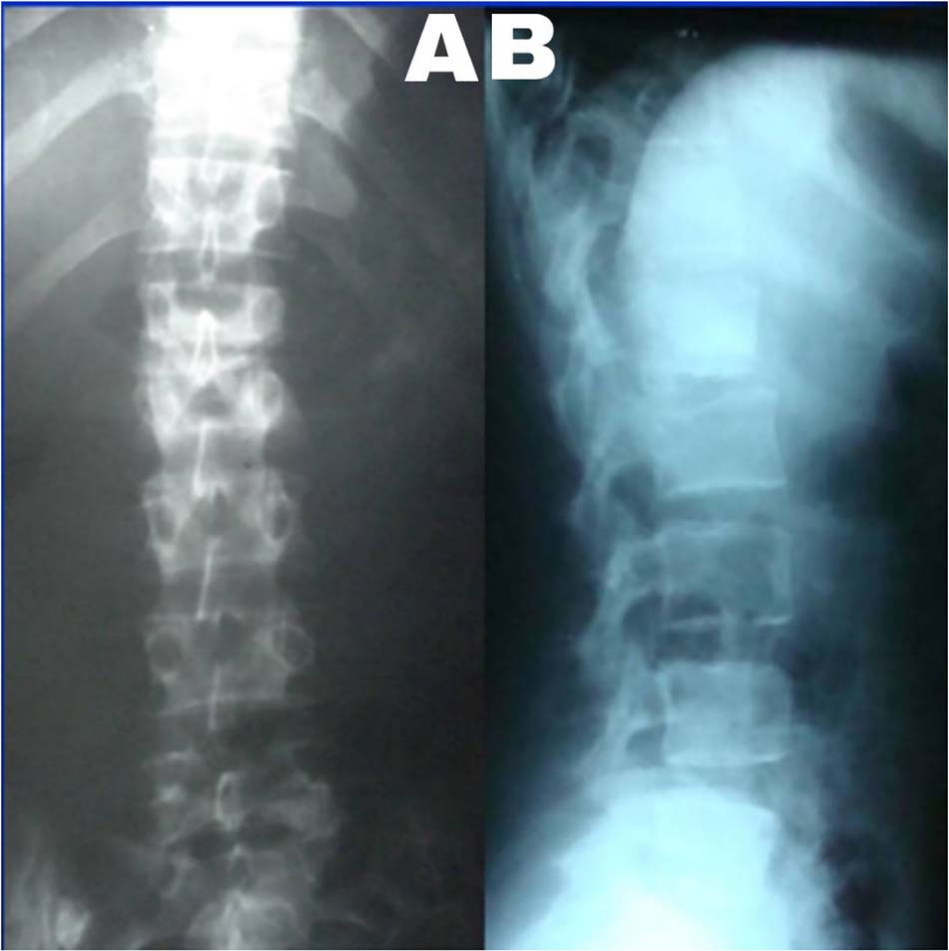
(A) Lumbar spine X-ray, anteroposterior view showed the collapse of the L1–2 intervertebral space. (B) Lumbar spine X-ray and lateral view showed L2 superior endplate erosion.
